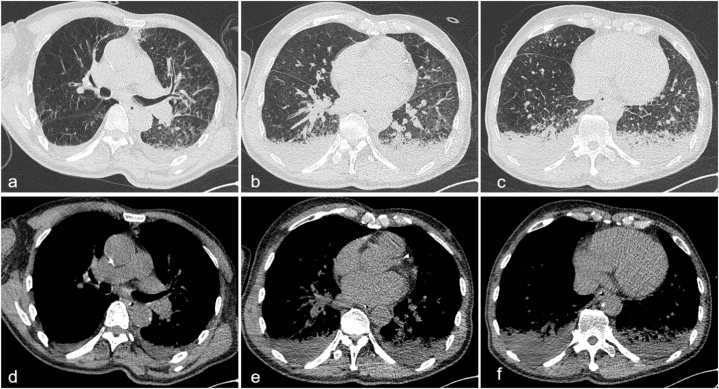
Emergency chest CT scan on the second day after sintilimab administration. (a–c) The lung window images. (d–f) The mediastinal window images. CT, computed tomography.
